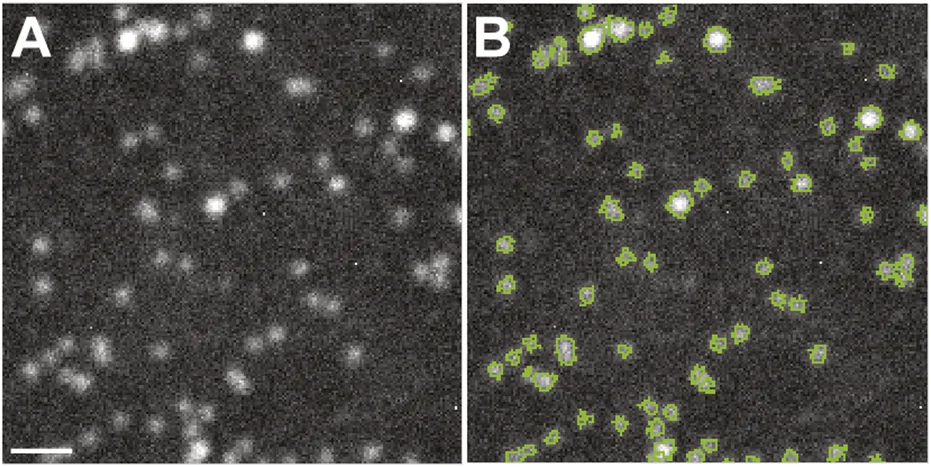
Expression levels of PSMA in patient cells collected from a peripheral blood draw of a metastatic castrate-resistant prostate cancer patient. (A) Raw image of PSMA labeling in CTCs. Magnification 63×. Scale bar equals 70 µm. (B) PSMA segmentation based on stationary wavelet transform, active contour, and watershed transformation (Matov, 2024h). The edges of the segmented PSMA areas are displayed in green.
